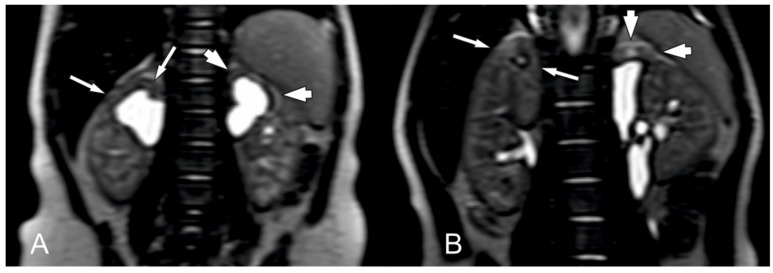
An example of MRI findings in T2-weighted image in two patients ((A) patient Nr 5R, 5L; (B) patient Nr 12) with bilateral duplex kidneys with suspected segmental dysplasia. (A) The parenchymal signal of upper poles in both kidneys is increased (lower extent in right kidney—long arrows), and parenchymal thickness and CMD are reduced (more advanced in left kidney—short arrows). (B) The parenchymal signal of upper pole of the right kidney is inhomogeneous and increased, parenchymal thickness is slightly thinner, and CMD is reduced (long arrows). The upper pole of the left kidney presents significantly reduced parenchymal thickness, increased parenchymal signal, and significantly reduced CMD (short arrows).
